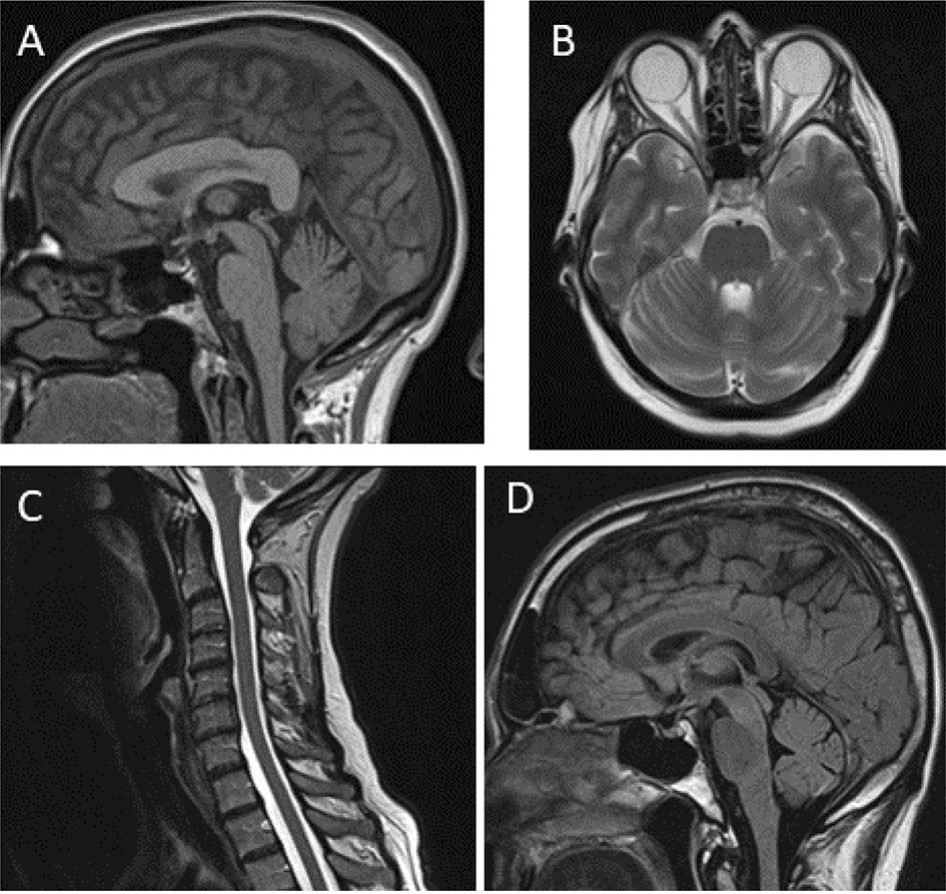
MRI sequences of Subjects #1-#3. MRI of the subjects did not show any signs of cerebellar or cervical spinal cord atrophy (exemplary sequences). A midsagittal T1-weighted image of cerebellum, subject #1. B axial T2-weighted image of cerebellum, subject #2. C sagittal T2-weighted image of cervical cord, subject #1. D midsagittal T2/FLAIR-weighted image of cerebellum, subject #3
